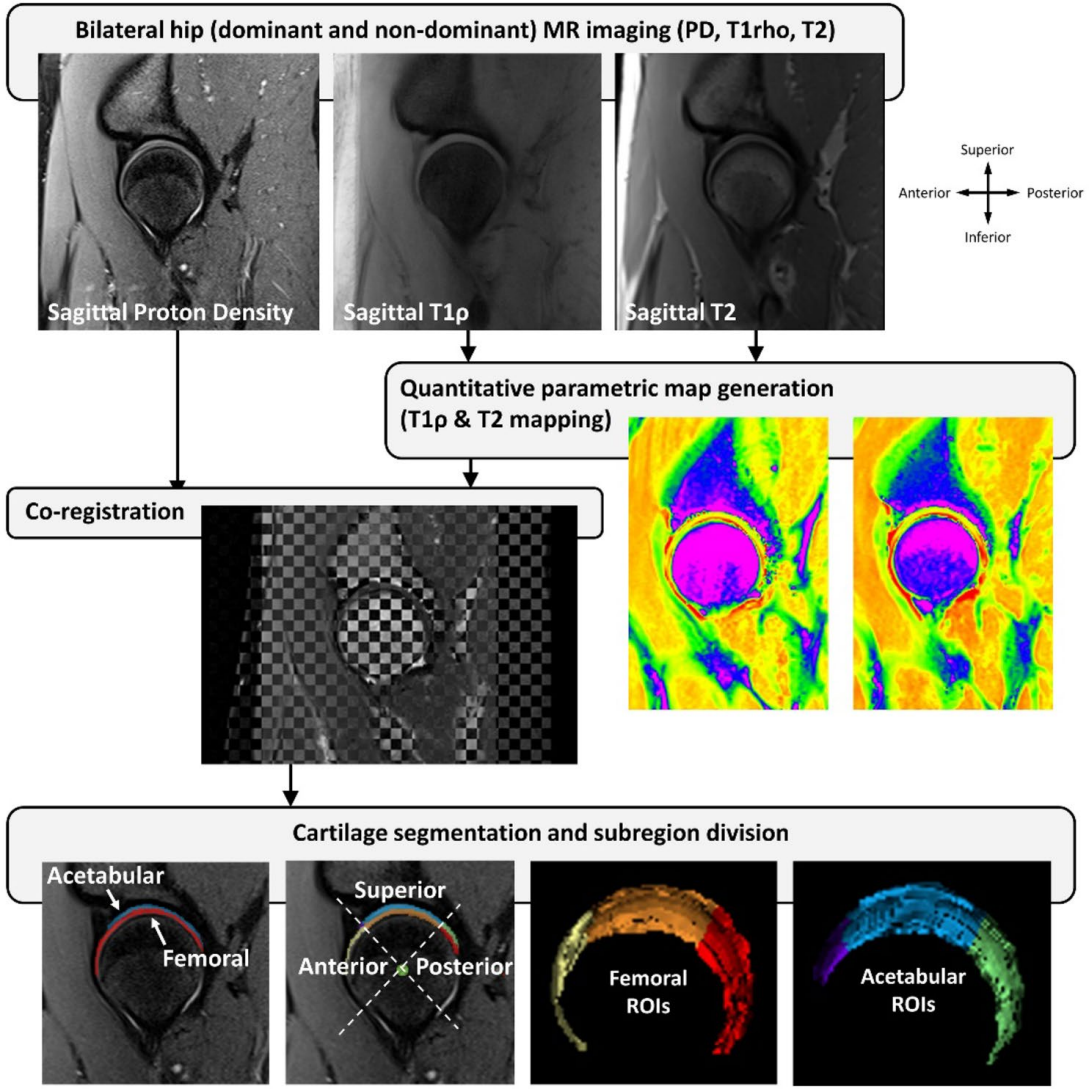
Assessment of cartilage composition on MRI scans. Cartilage T1ρ and T2 maps were generated and co-registered with the sagittal proton density image on which the femoral and acetabular cartilage compartments were segmented. T1ρ and T2 relaxation times were computed within the entire femoral and acetabular mask as well as the anterior, superior, and posterior subregions.
